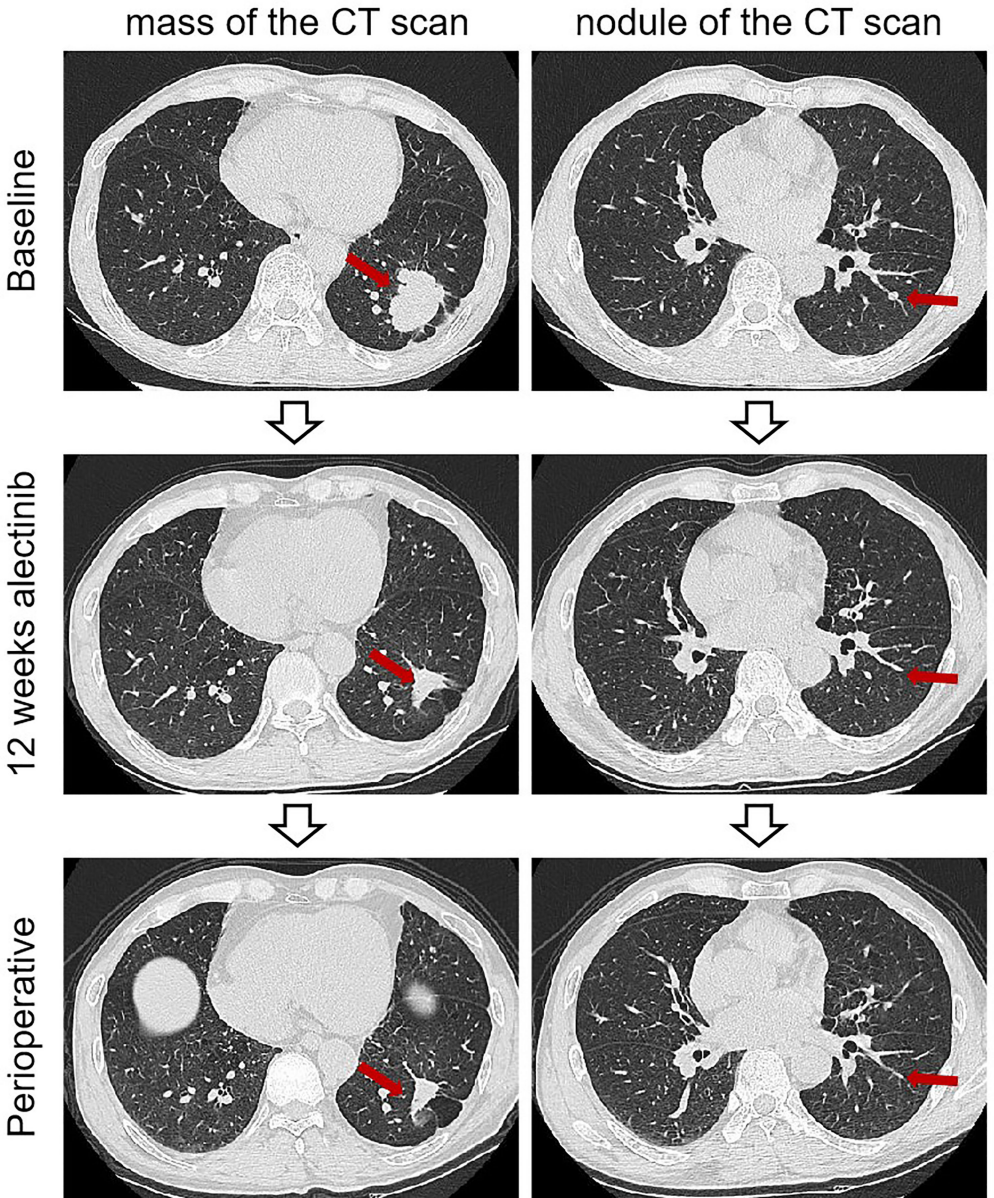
Imaging findings of case 1. The computed tomography scan of before and after neoadjuvant alectinib. CT, computed tomography.
